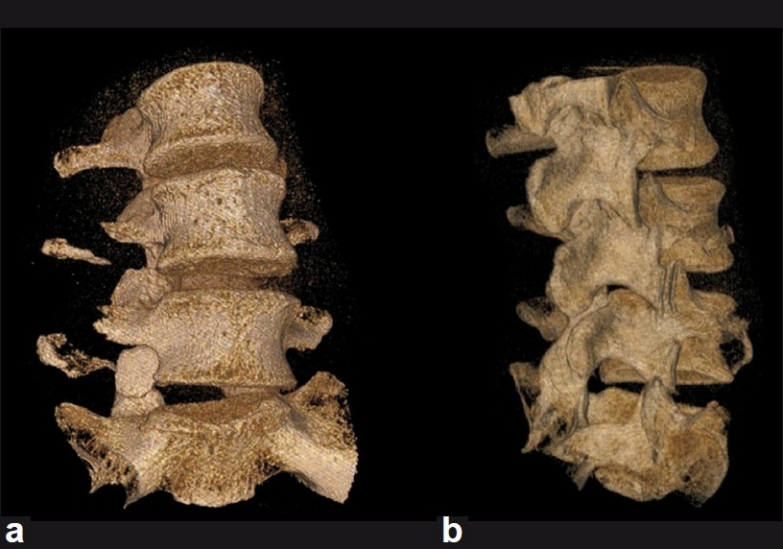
Anterior (a) and right lateral (b) views of the three-dimensional reconstruction of the computed tomography scan of the lumbar spine, demonstrating the unilateral perched facet joint and asymmetric widening of the L5–S1 disc space
